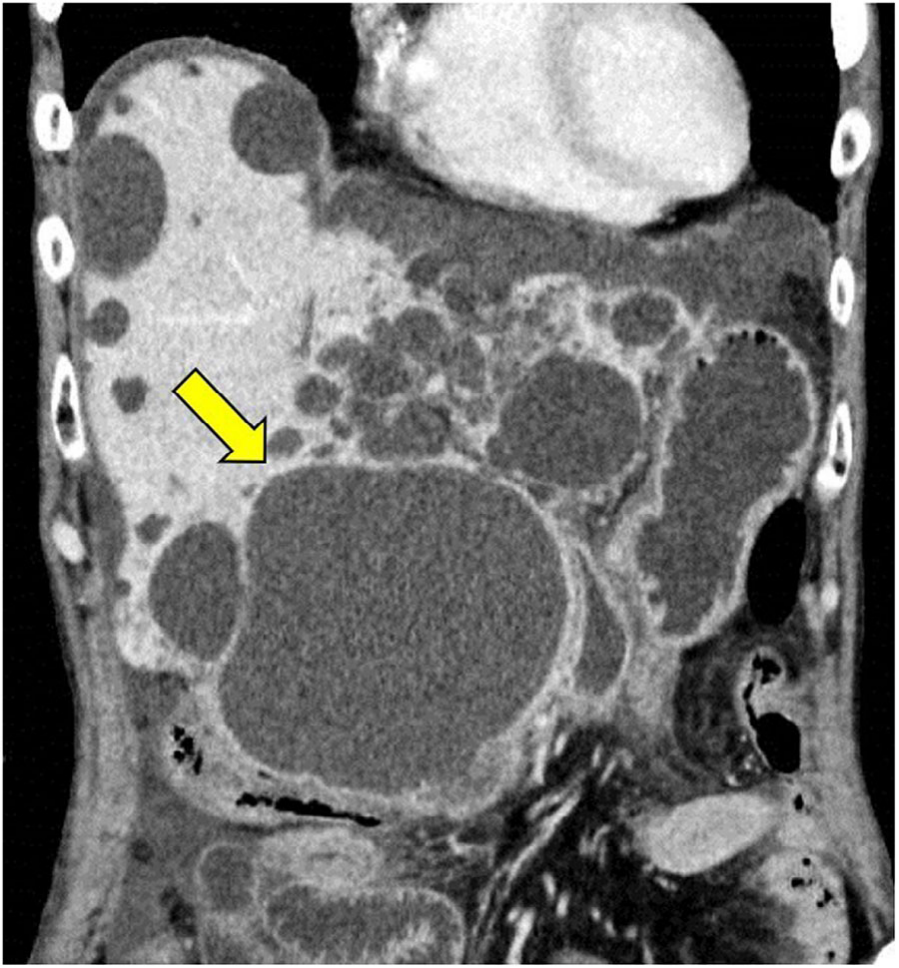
Contrast‐enhanced computed tomography reveals a 121‐mm cyst in the caudate lobe of the liver (arrow). The cyst wall is thick, suggesting infection. A small amount of ascites is seen on the liver surface.
